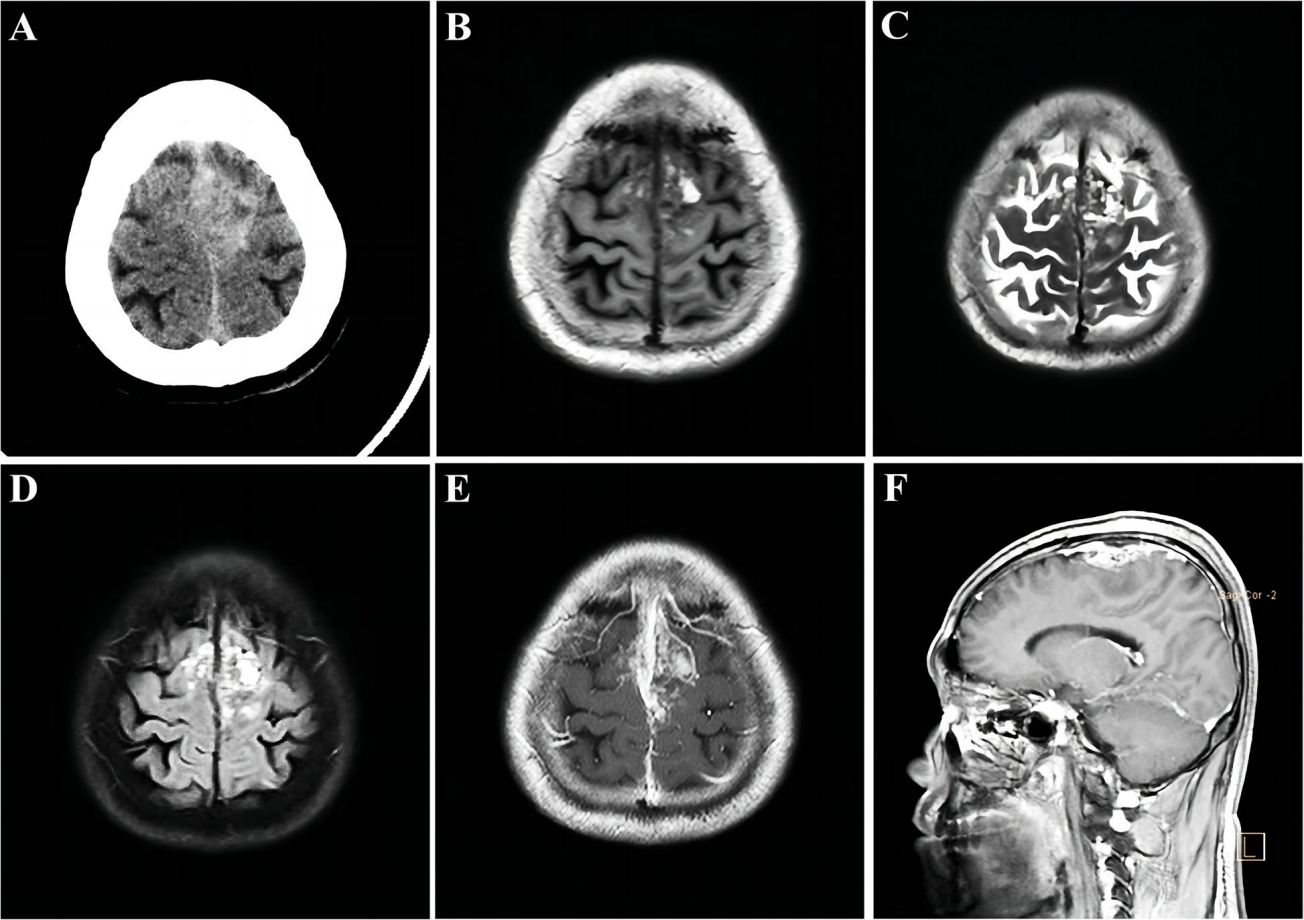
A Admission CT showed bilateral parasagittal high-density lesions, mainly on the left side, located in front of the motor area of the brain. B T1-weighted images and (C) T2-weighted image showed a parasagittal mass with irregular mixed signals, and some speckle high-signals was seen in it. D T2-FLAIR image showed mixed signals with ill-defined borders, encompassing the superior sagittal sinus, and invading the adjacent skull. E Axial T1-weighted images with contrast enhancement. F Sagittal T1-weighted image with contrast enhancement, showed uneven and obvious enhancement of the lesion, involving bilateral parasagittal dura and sagittal sinus, and local brain tissue compression
